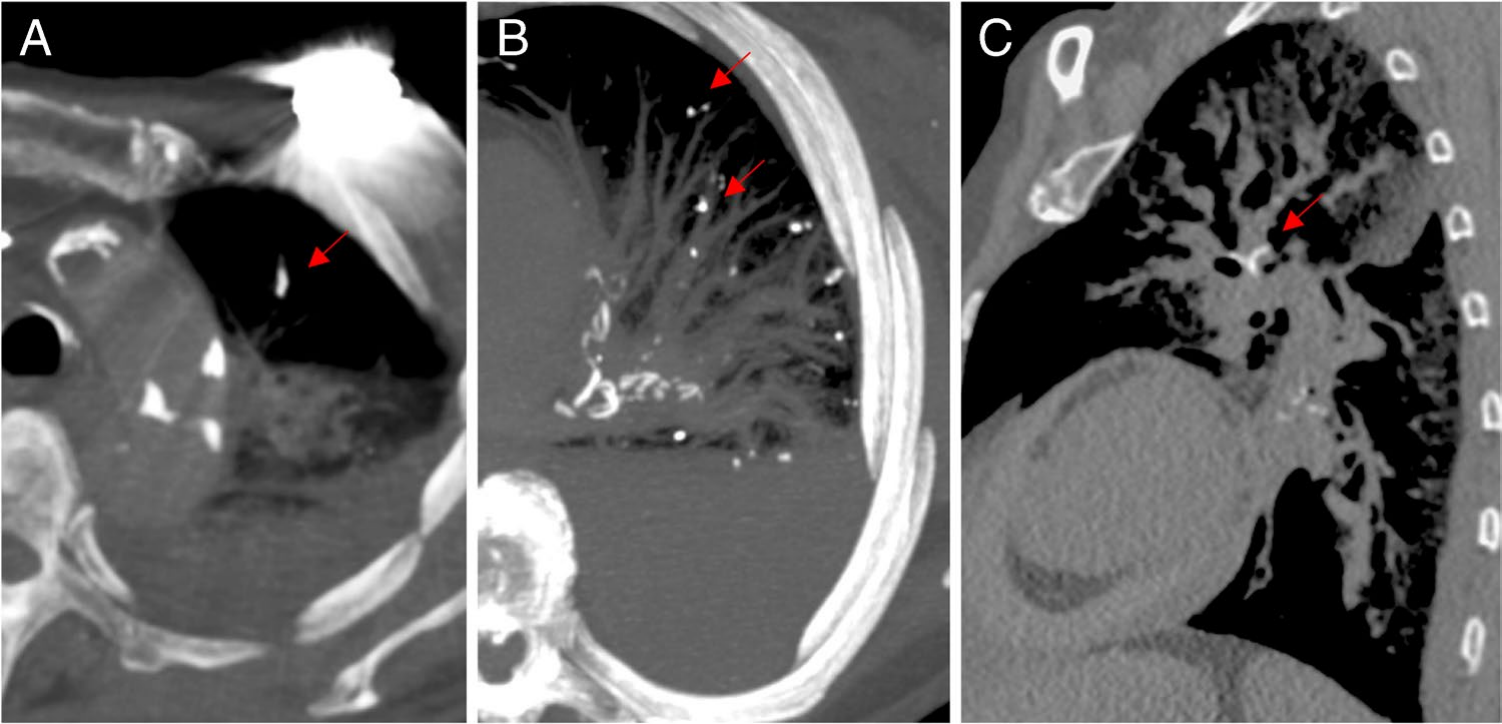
Exemplary chest CT images (bone window) with hyperdense structures classified as grade 3 pulmonary cement (PCE). A Axial reformat with signs of a slightly branched PCE. B Axial reformat with characters from multiple PCEs. C Sagittal reformat with a branched PCE. The red arrows point to the PCE
